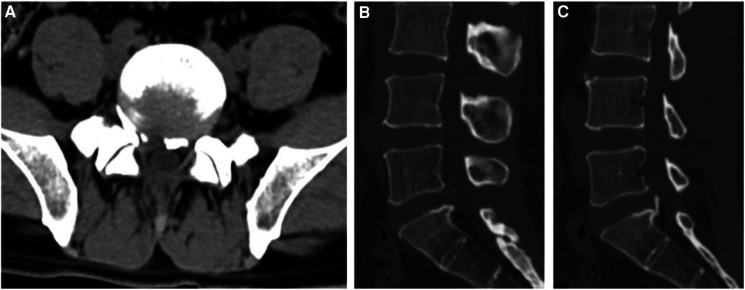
Postoperative CT images: (A) transverse CT image of the patient in figure 2 after the operation shows that the bone fragment that compressed the nerve root on the right side has been removed, (B) a sagittal CT scan shows that the right bone fragment has been completely removed, (C) the sagittal CT scan shows that the left bone fragment is firmly connected to the vertebral body and is not resected during the operation.
